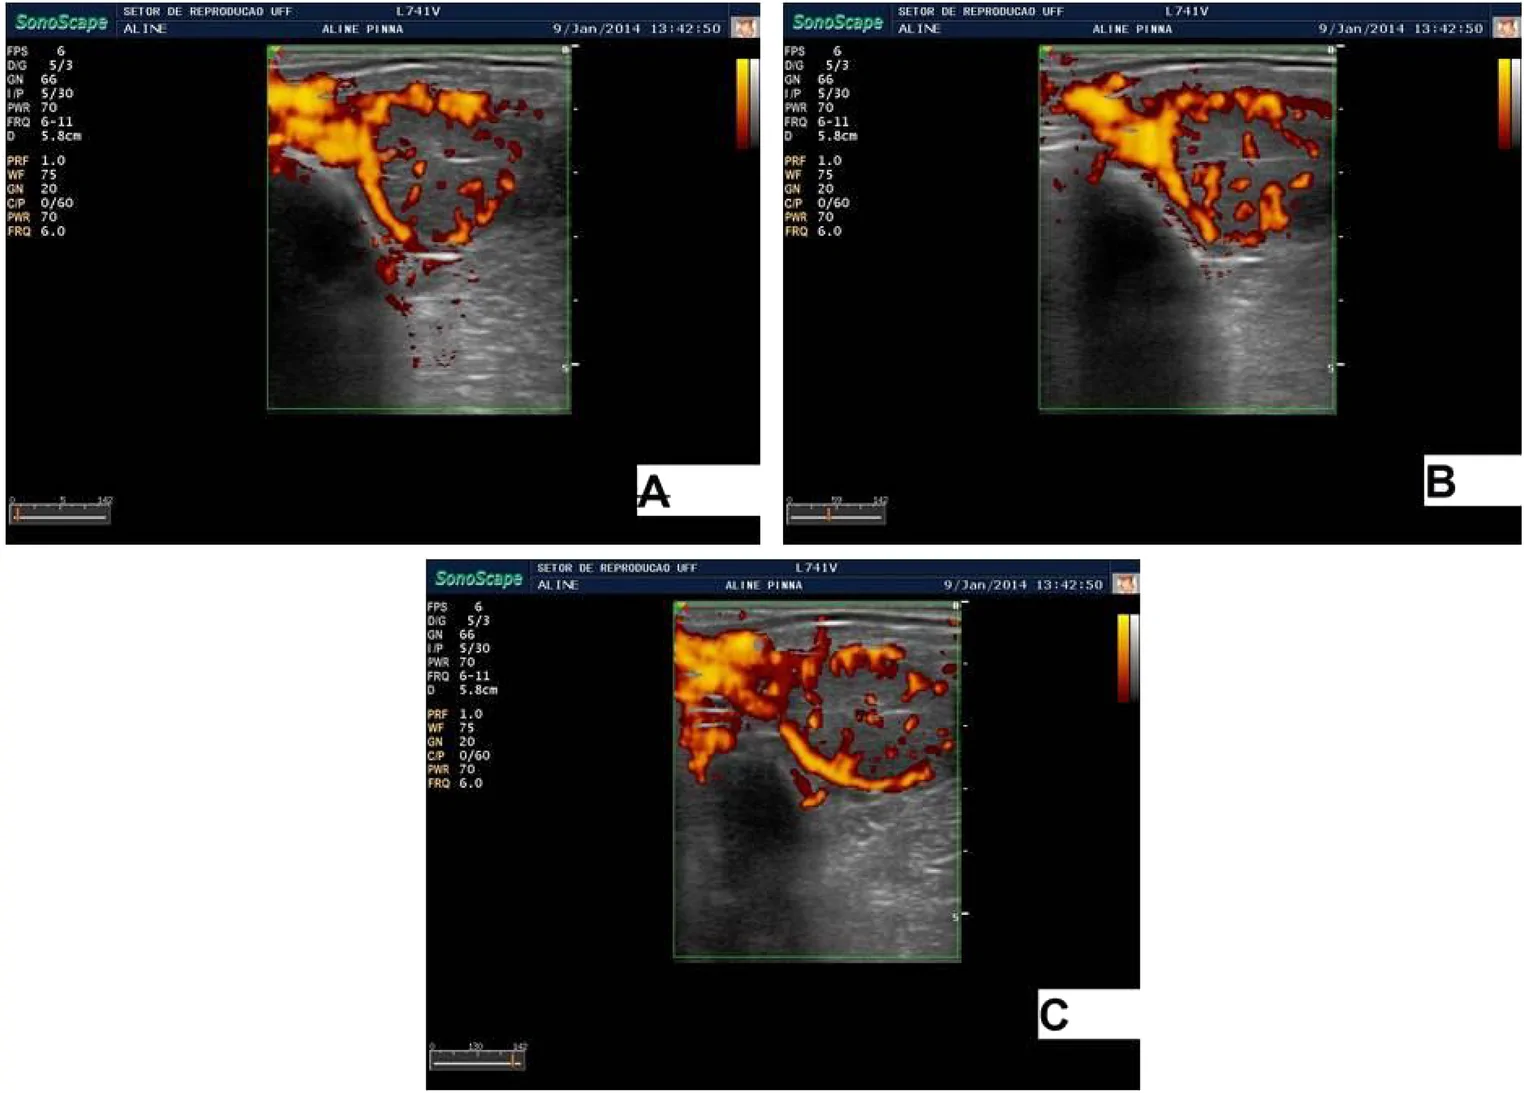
Capture of the most representative corpus luteum images obtained by power Doppler ultrasonography. (A, B, C) Frozen frames from a video representing the largest luteal areas containing the greatest amount of color signals
